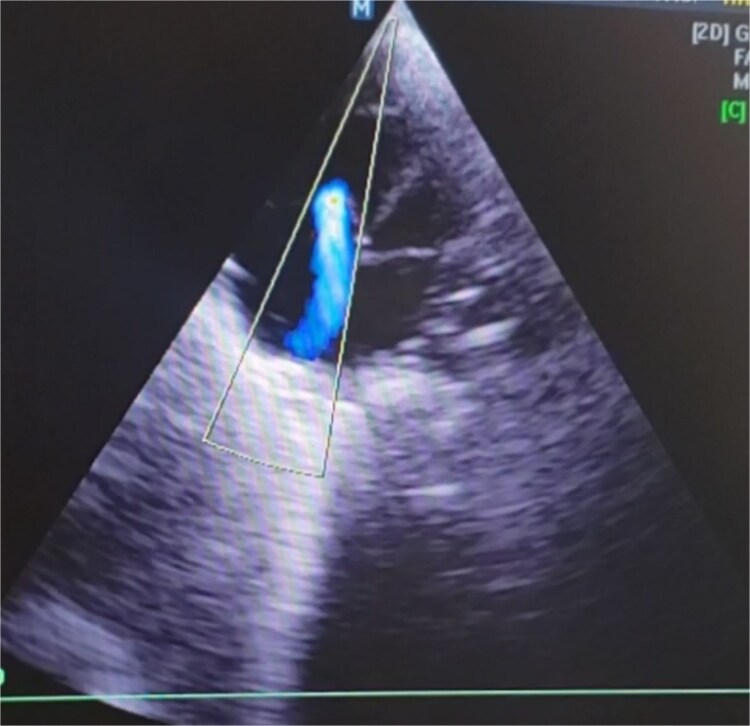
The echocardiographic image demonstrates severe tricuspid regurgitation. This pathological finding is usually associated with severe PPHN.
